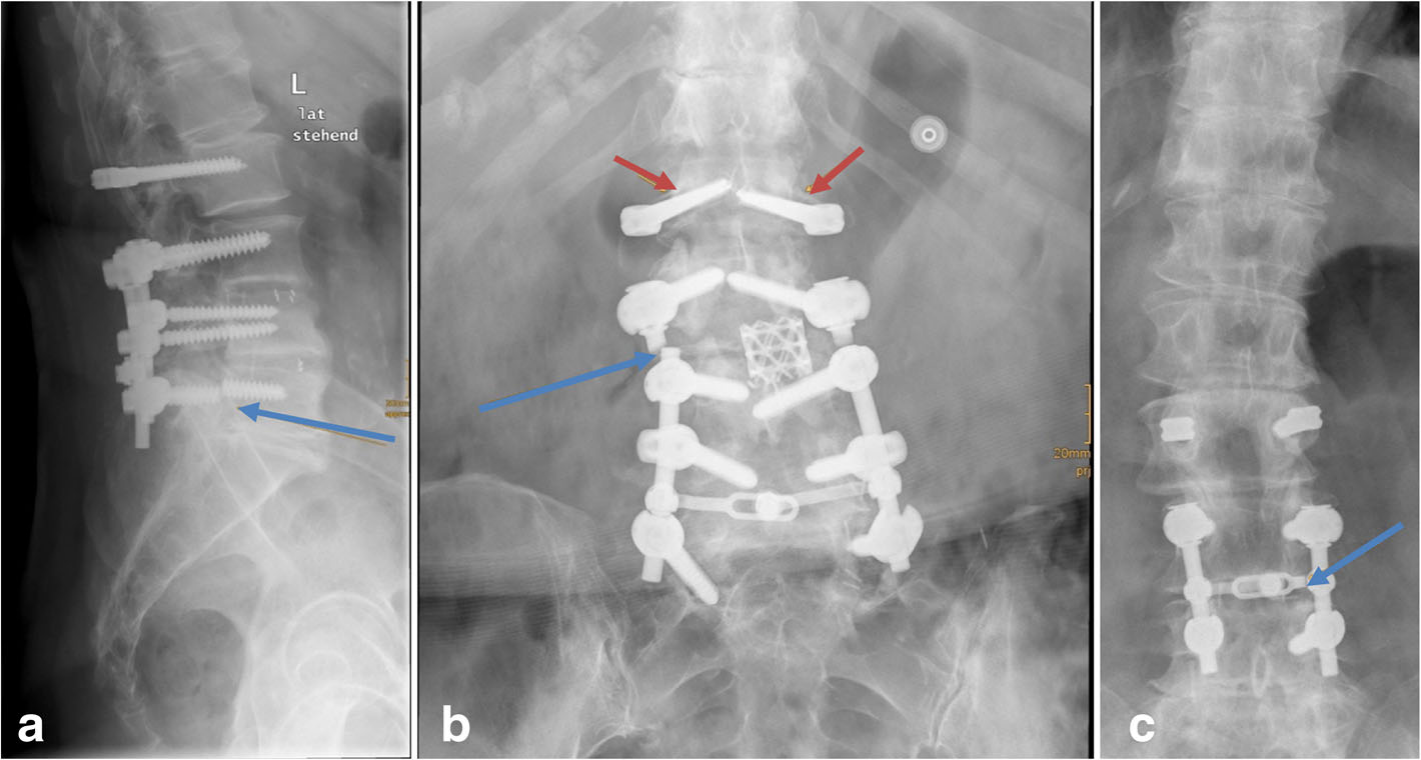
Radiographs representing the three types of observed implant failure: (a) breakage of the left inferior pedicle screw (blue arrow); (b) breakage of the titanium rod inferior to the dynamic instrumented level (blue arrow) and loosening of the Dynesys screws (red arrows); (c) breakage of the crossbar (blue arrow)
